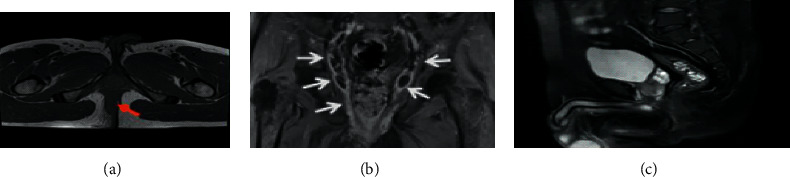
MRI images of a 48-year-old male patient. (a) Transverse axial enhanced scanning; (b) transverse axial T2WI sequence; (c) ultrasound images.
